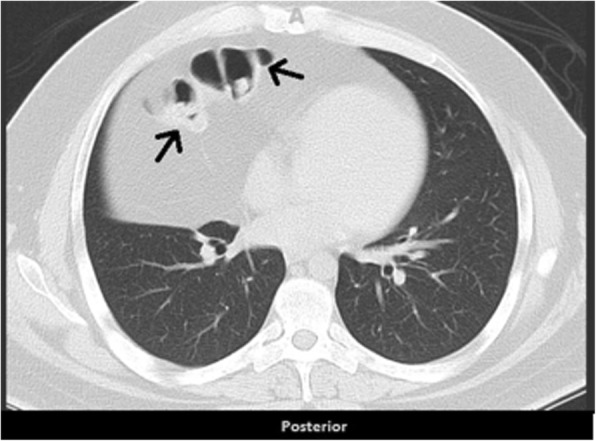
Computed tomographic scan (transverse section) showing bowel loops (*arrows*) and omentum herniating through the right side of the diaphragm
